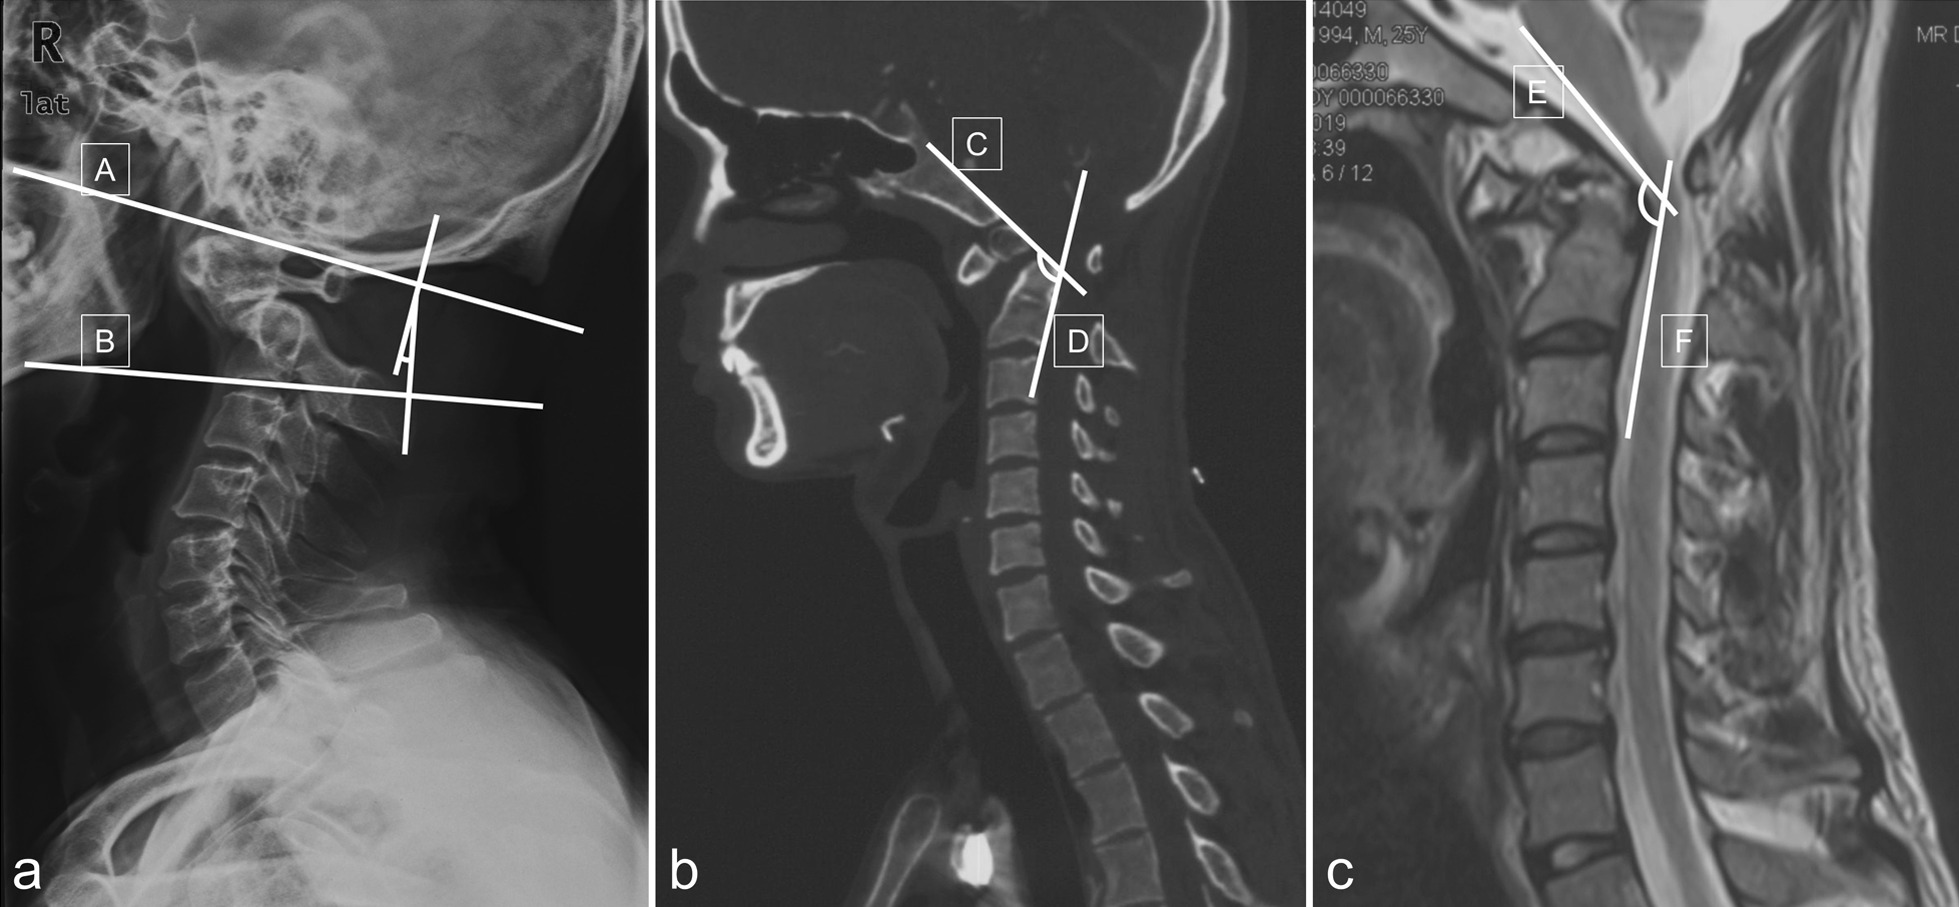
Measurement of the O-C2A, CCA and CMA. a O-C2A: Angle between McGregor line (A line) and the line (B line) tangential to the inferior aspect of the axis. b CCA: Angle of the line of inferior 1/3 clivus (line C) and the line extending from the posterior border of the dens to the posteriorinferior border of the axis body (line D). c CMA: Angle of the line extending the anterior border of the ventral medulla (line E) and the line extending the anterior border of the ventral upper cervical spinal cord (line F) in midline sagittal T2 MRI. O-C2A, Occipitoaxial angle; CCA, clivus-canal angle; CMA, cervicomedullary angle
